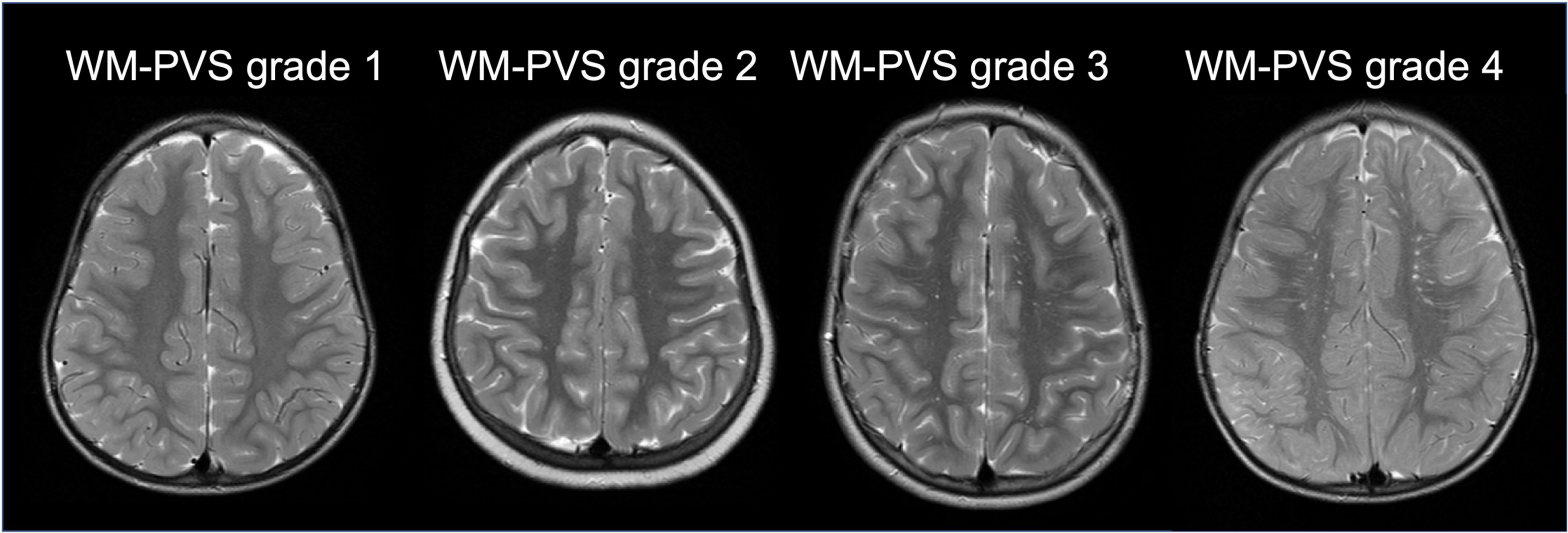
WM-PVS grades. Representative cases of the four WM-PVS grades observed in our cohort. WM-PVS on T2-weighted axial images appear as hyperintense structures in the white matter, linear or punctate depending on whether their course is parallel or perpendicular to the axial acquisition plane, respectively.
